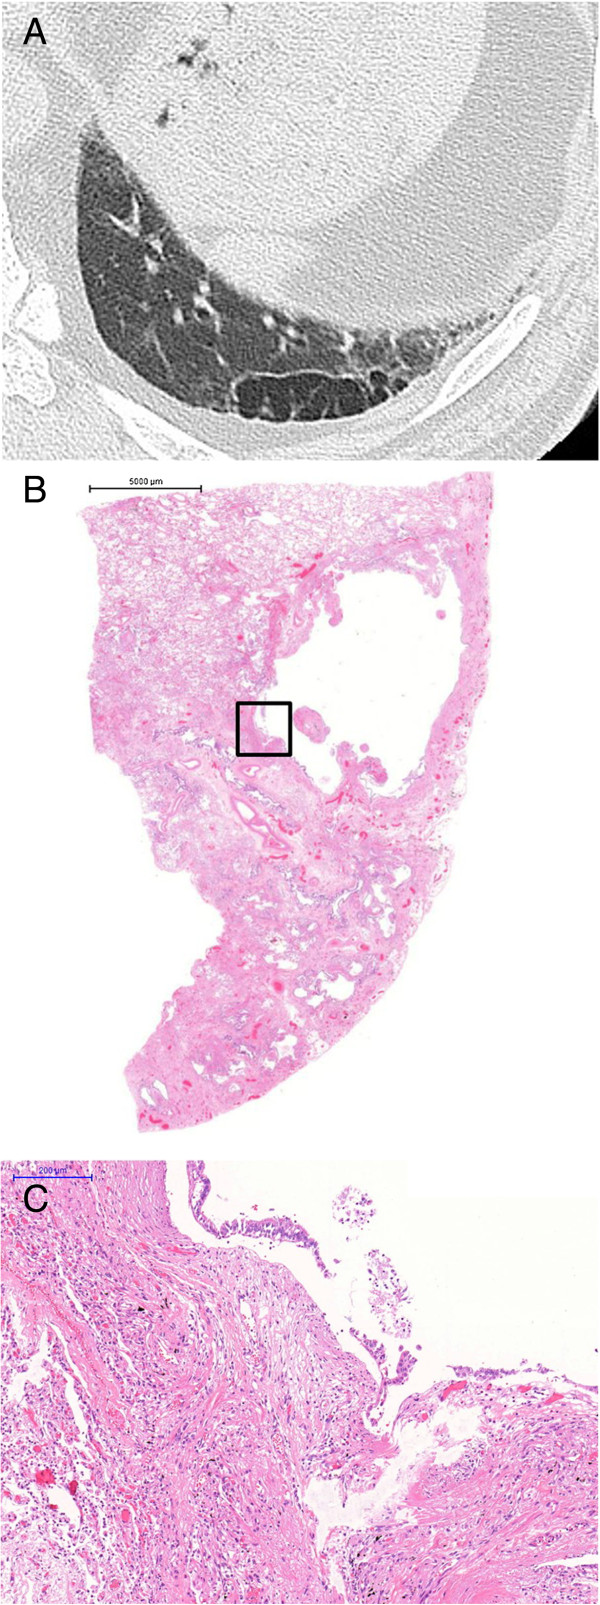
Images of a CPFE patient with lung cancer in the right lower lobe who died from a non-respiratory cause. (A) CT scan performed within 1 month of death; view of the left lower lobe showing TWCLs with slight reticulation on the pleural predominance. (B) Paramount view showing pathological TWCLs with fibrosis in the left lower lobe; corresponds to the CT scan (Figure 3-A). TWCLs involving the bronchiole and parenchyma with a dense fibrous wall beneath the terminal bronchioles. (C) High-power view of the square lesion in Figure 3-B showing fibroblastic focus in the fibrous walls.
